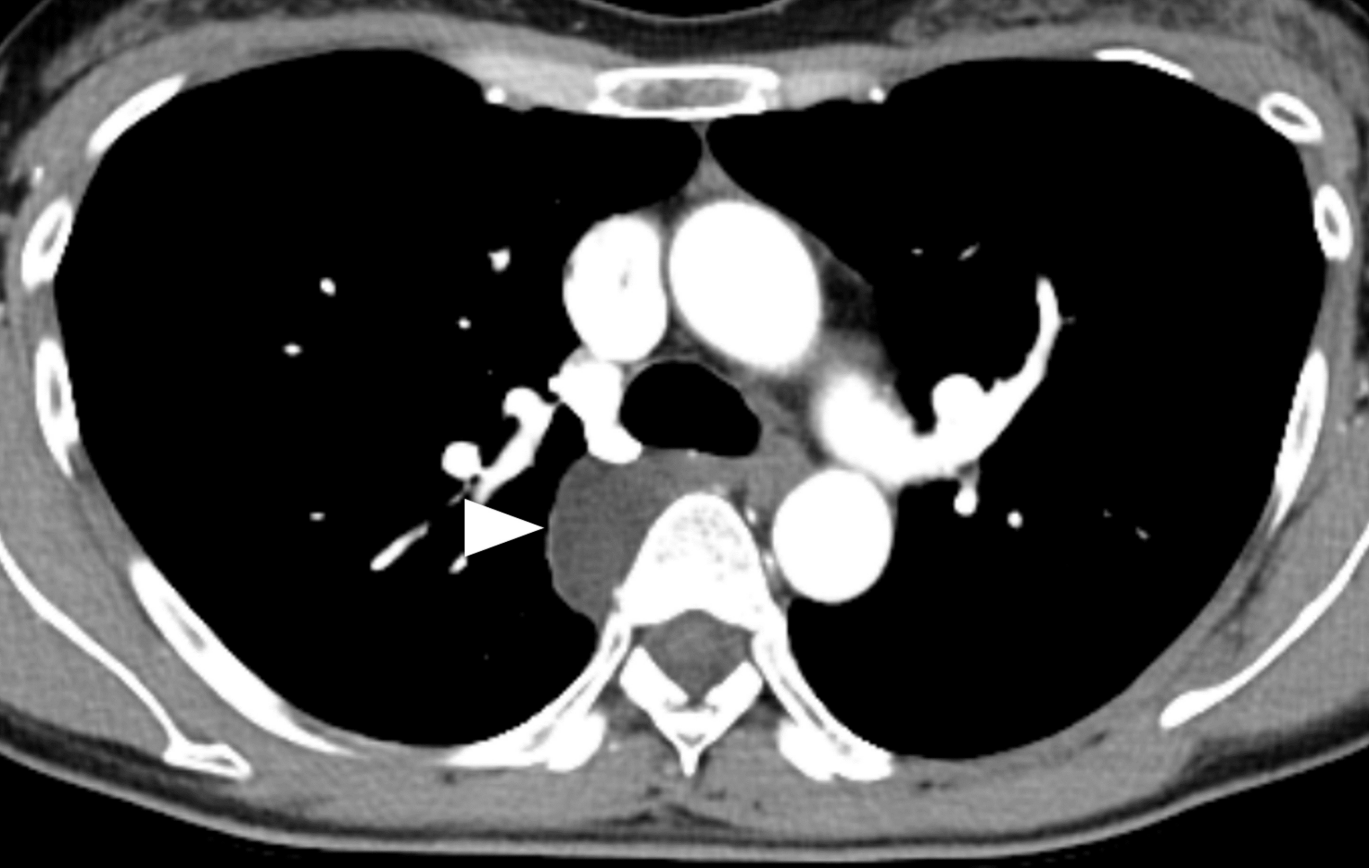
Contrast-enhanced computed tomography (CECT) Contrast-enhanced axial CT image showing a well-defined, non-enhancing lesion along the right side of the vertebral body (arrowhead).
